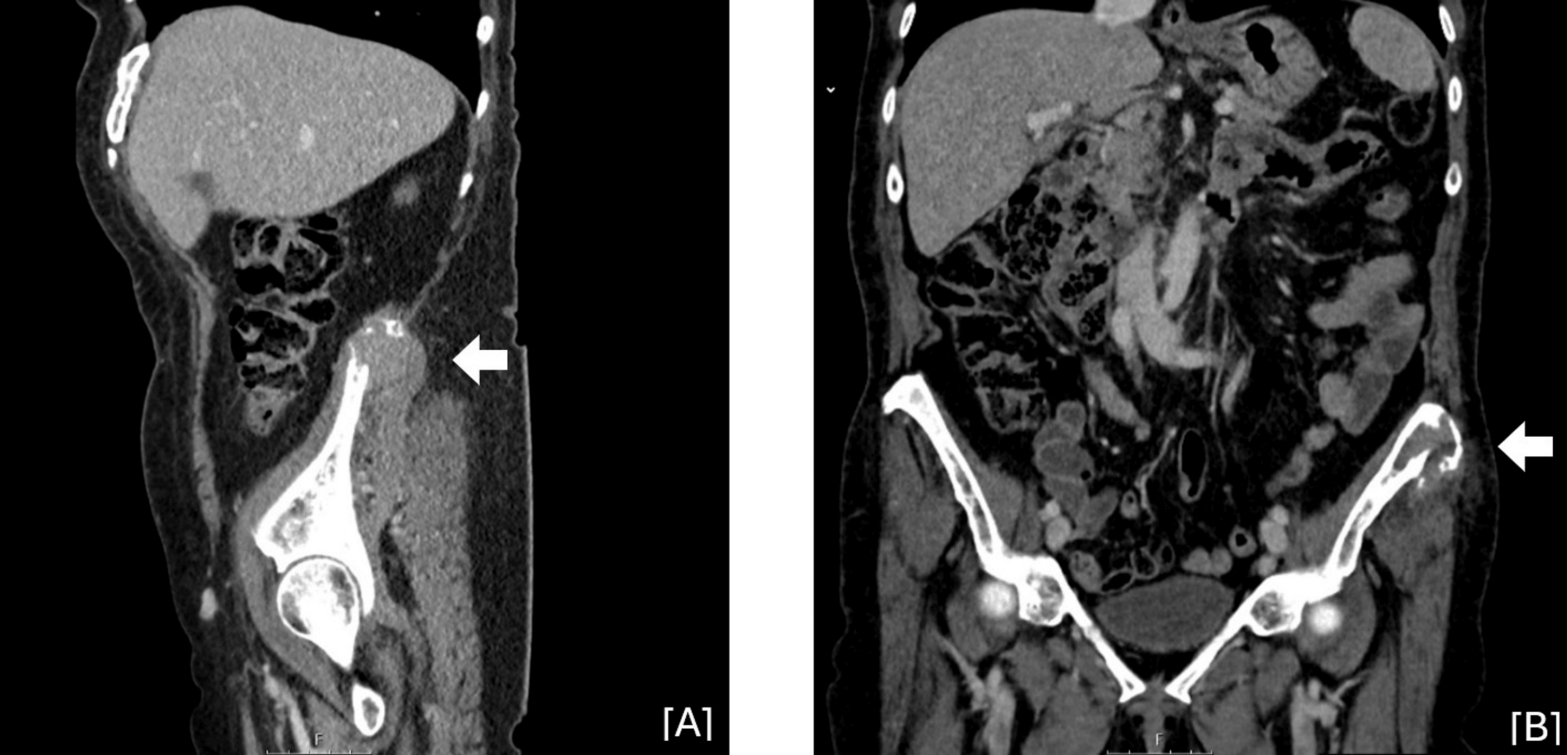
Abdominal and Pelvic Computed Tomography A large bone lesion with a soft tissue component on the right iliac wing (A, white arrow) measuring approximately 56 mm and another lesion on the left anterior superior iliac spine (B, white arrow) measuring approximately 32 mm
